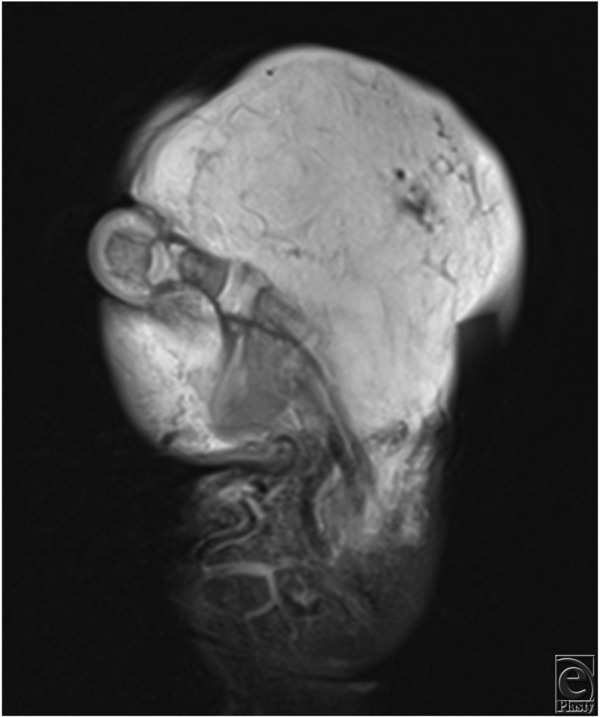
T1-weighted magnetic resonance image of the patient's right hand.
